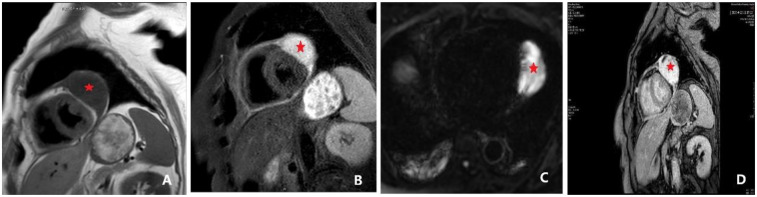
Cardiac magnetic resonance (CMR) findings, including late gadolinium enhancement (LGE). (A) Cardiac MRI revealing a space-occupying lesion within the pericardium, lateral to the left ventricle, suggestive of a hemangioma. The findings also included a thickened pericardium with a small amount of effusion. An ovoid lesion with abnormal signal intensity (indicated by *), measuring approximately 37 × 62 × 61 mm, was identified in this location. The lesion appeared hypointense on T1-weighted imaging (T1WI), and a discernible fat plane was present between the lesion and the lateral wall of the left ventricle. (B) Lesion appeared hyperintense on T2-weighted imaging (T2WI). (C) Lesion appeared hyperintense on both diffusion-weighted imaging (DWI) and apparent diffusion coefficient (ADC) maps. Following contrast administration, the lesion demonstrated marked heterogeneous enhancement, with progressive internal fill-in observed on delayed scans. (D) Late gadolinium enhancement (LGE) demonstrating progressive internal fill-in.
